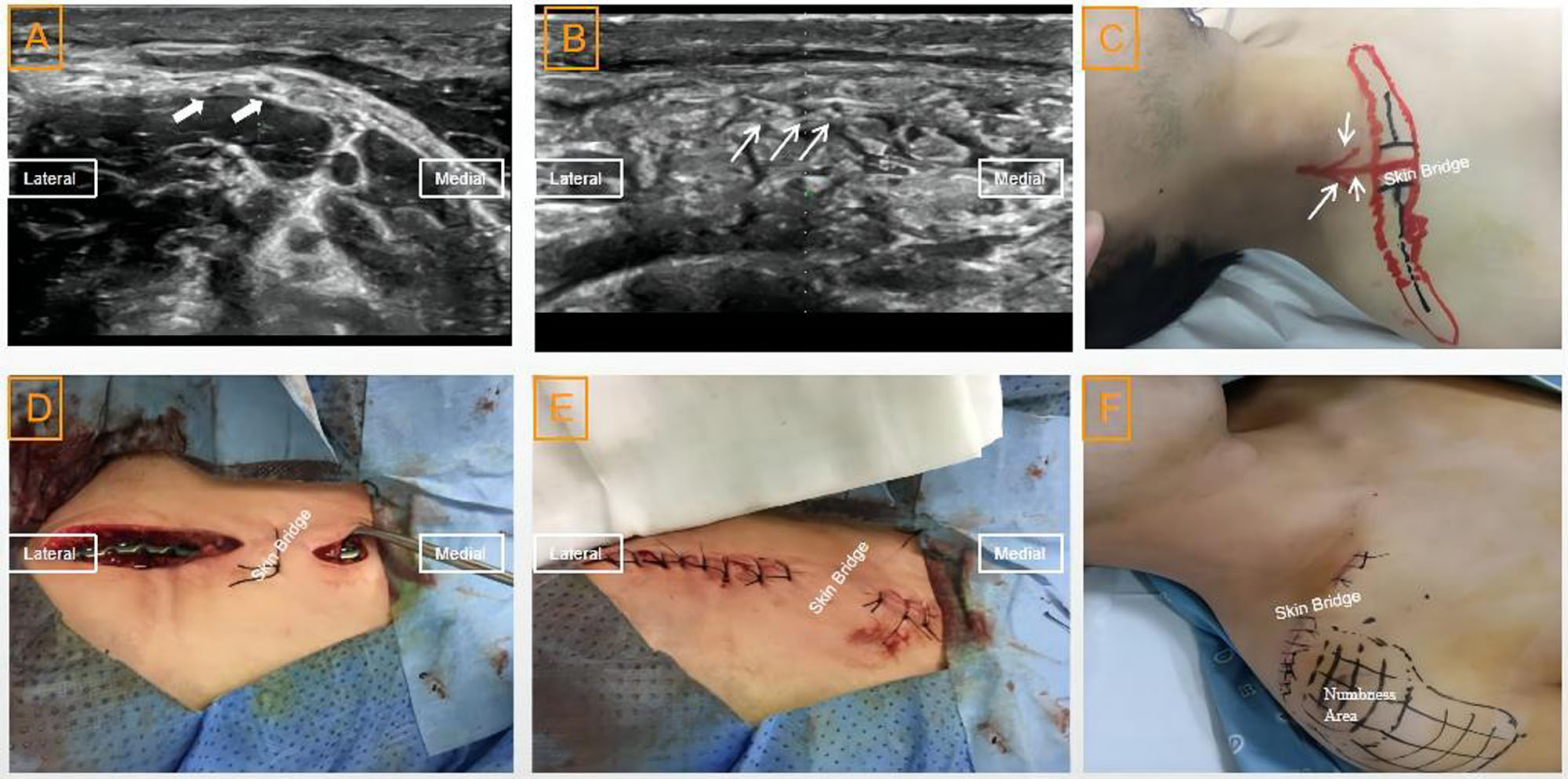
Ultrasound images, projection on body surface of the supraclavicular nerve, skin flap (bridge) and numbness area after the operation in case 3: (A) ultrasound images of the branches of the supraclavicular nerve at its origin (indicated with a thick white arrow); (B) ultrasound images of the branches of the supraclavicular nerve (indicated with a thin white arrow) anterior to the clavicle (indicated with a white cross arrow); (C) projection of the branches of the supraclavicular nerve on the body surface and skin flap (bridge) before the operation; (D) skin flap during the operation; (E) skin flap after the operation; and (F) numbness area after the operation.
